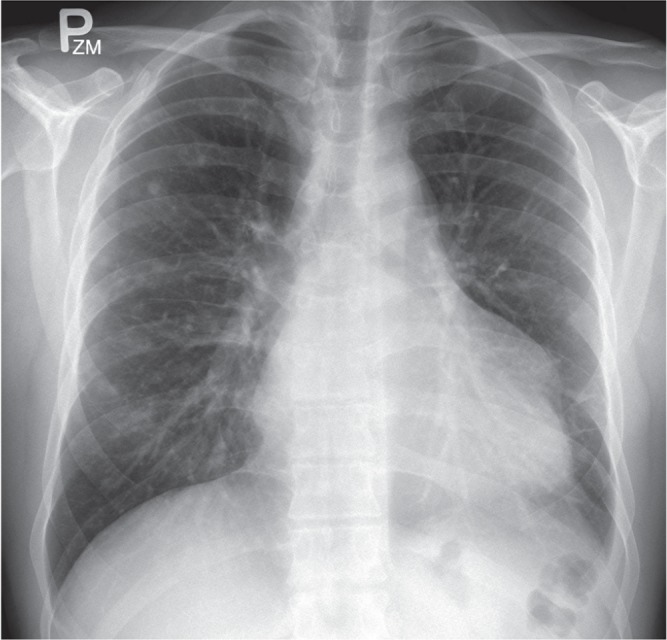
Plain chest x-ray shows abnormal left ventricular contour with silhouette sign. The lung parenchyma contains small pulmonary nodules, later proven to be pulmonary metastases. Blurred left hemidiaphragm and blunted costophrenic angle suggest pleural effusion.
